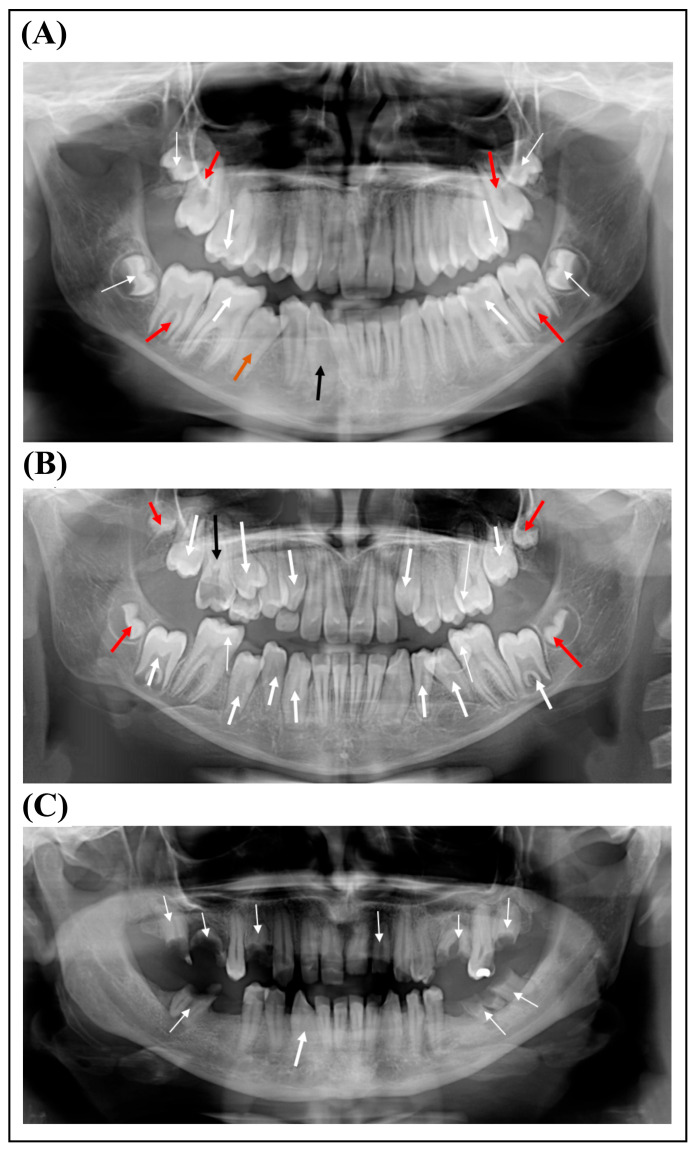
(A) Panoramic radiograph of the proband shows occlusal caries (thick white arrows), delayed permanent teeth eruption of the second molars (red arrows) and rotation of tooth 43 (thin black arrow). Thin white arrows indicate the unerupted third molars, and the orange arrow indicates the second premolar being erupted. (B) Panoramic radiograph of the proband’s sister shows several teeth (13, 14, 23, 34, 35, 43, 44 and 45) in the process of eruption (thick white arrows). Thin white arrows indicate dental caries, and black arrow indicates root remnant of tooth 16 and red arrows indicate the unerupted third molars. (C) Panoramic radiograph of the proband’s mother. Thin white arrows indicate root remnants and the thick white arrow indicates the rotation of tooth 43.
